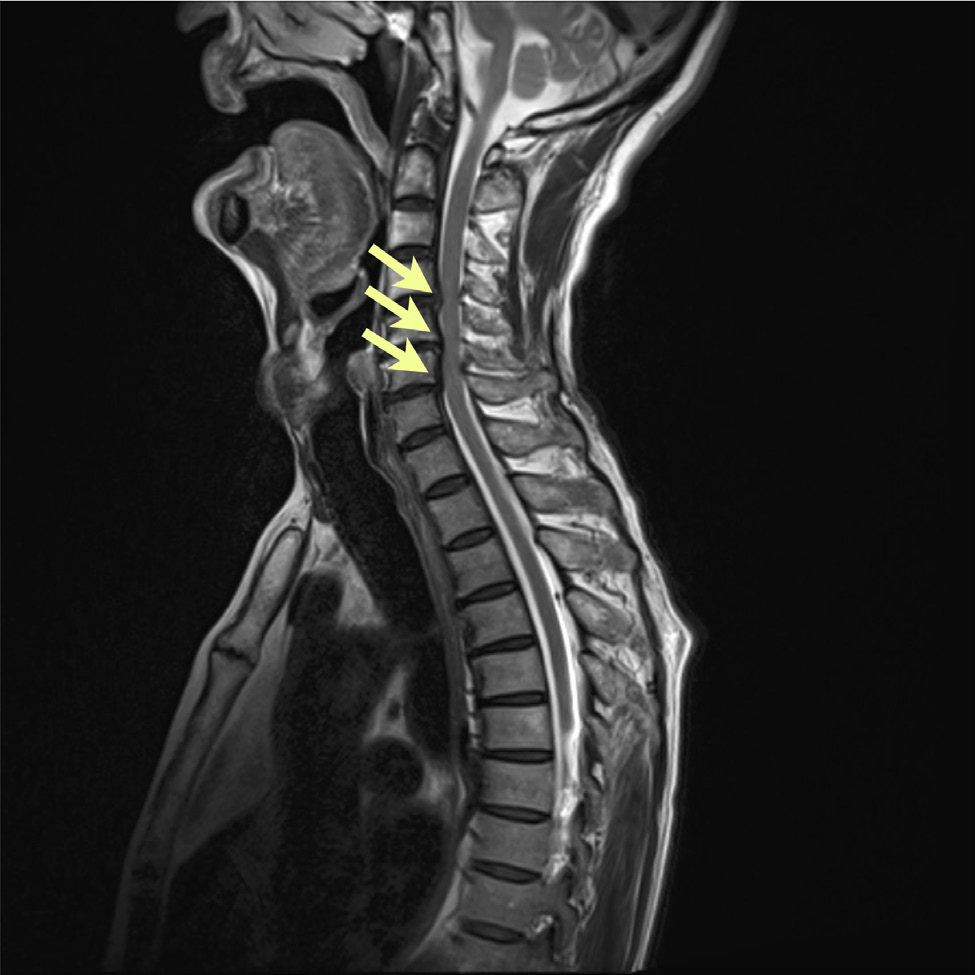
MRI Sagittal image of the spine. T2-weighted MRI shows mild cervical stenosis at C4–5, C5–6, and C6–7 levels (arrows).
